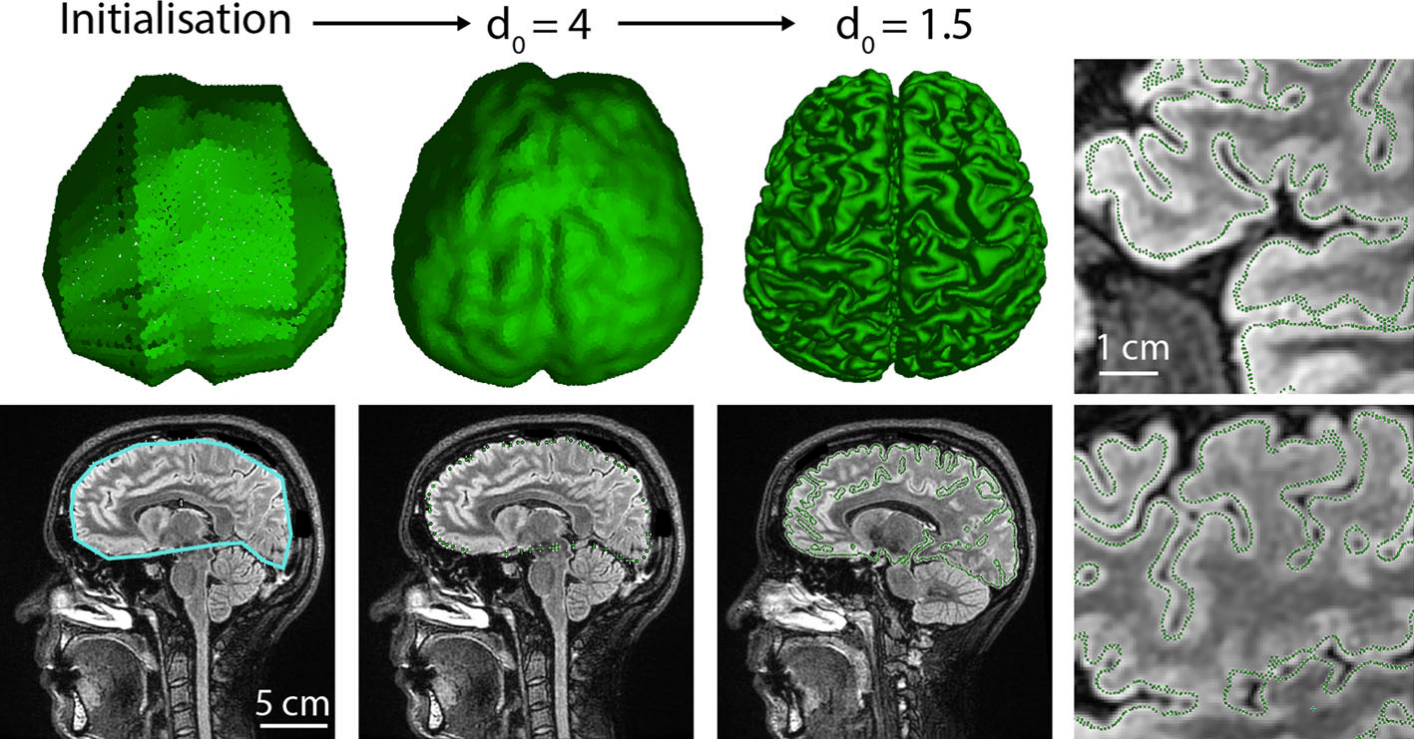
Human brain MRI surface segmentation. From left to right: 1 - initialization of the shape with ROI skeleton (blue line on the data image). 2 - After segmentation convergence with *d*_0_=4, many details of the cortex are missed. 3 - Segmentation refinement by decreasing *d*_0_ to 1.5. 4 - Zooms showing details being retrieved by the finest segmentation where surfels appear as green dots
